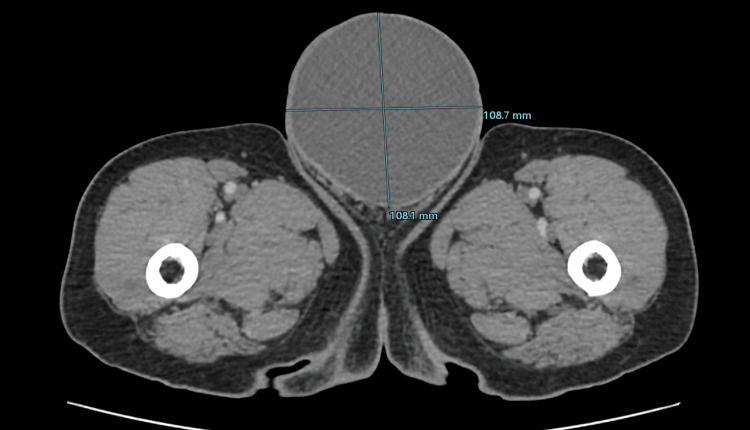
Axial computed tomography image of the scrotum demonstrating a large, well-circumscribed cystic lesion measuring approximately 10.8 × 10.9 cm, causing marked scrotal enlargement without evidence of local invasion.
